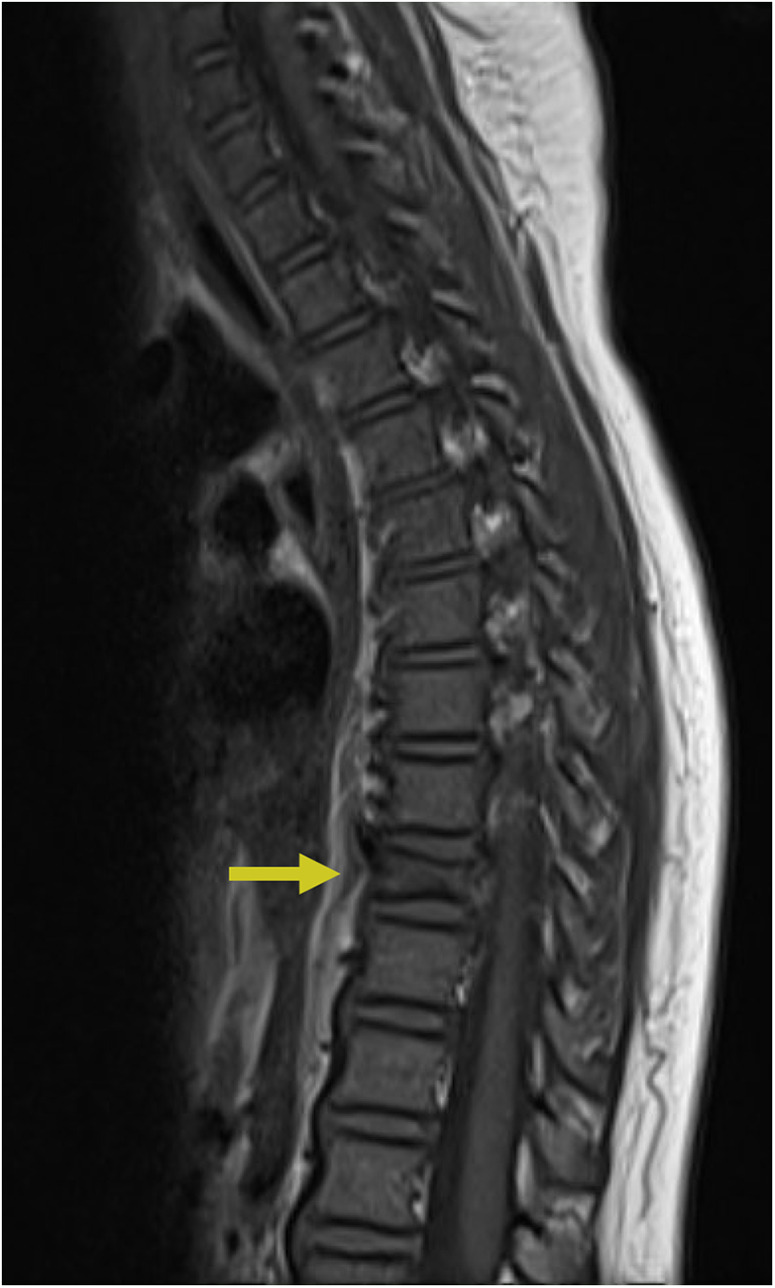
Congenital butterfly vertebra. Sagittal T2 MRI of the lumbar spine demonstrating a congenital T9 thoracic butterfly vertebra.
